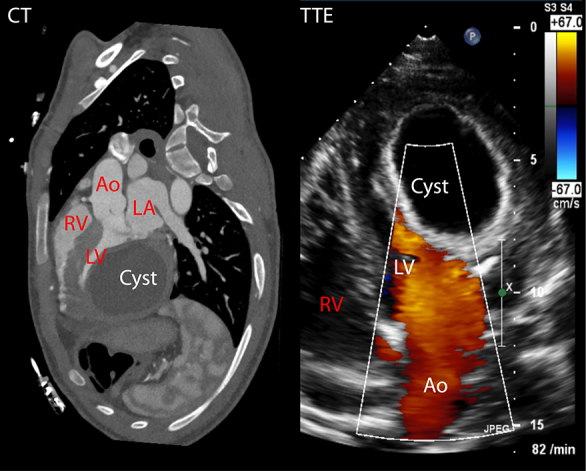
CT and TEE: intramyocardial LV cyst with thinned wall, narrow inflow/outflow.
